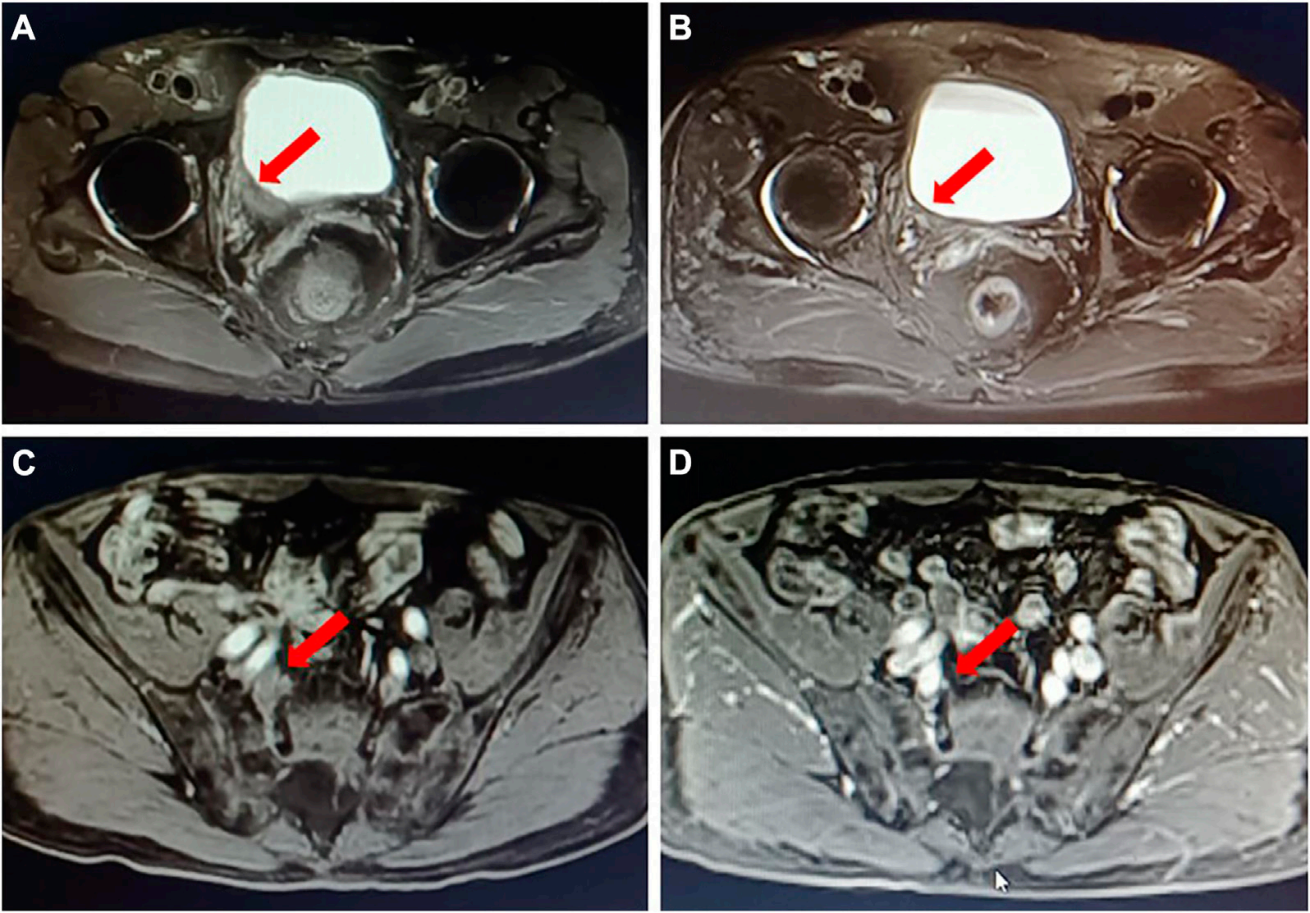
A typical case of neoadjuvant therapy of DV in combination with an immune checkpoint inhibitor. A 72 years old male patient with “gross hematuria” was admitted; MRI showed that a bladder tumor in the right wall of the bladder with the inner segment of the right ureteral bladder wall involved (A); multiple mildly enlarged lymph nodes paravascular were found on the right iliac with a maximum of 1.1 cm, and lymph nodes metastasis were considered (C). Pathology of biopsy confirmed bladder high-grade urothelial carcinoma, with PD-L1 low expression (IHC) and HER2 (2+) (IHC). DV 2 mg/kg plus toripalimab 3 mg/kg, Q3w was given as neoadjuvant therapy for three circles. Then MRI was performed to evaluate the outcomes of the neoadjuvant treatment; it showed a radiological complete response (B, D). After laparoscopic right pelvic lymph node dissection with partial bladder incision and right ureteral bladder replantation, the postoperative pathology confirmed no evidence of cancer, suggesting a pathological complete response.
